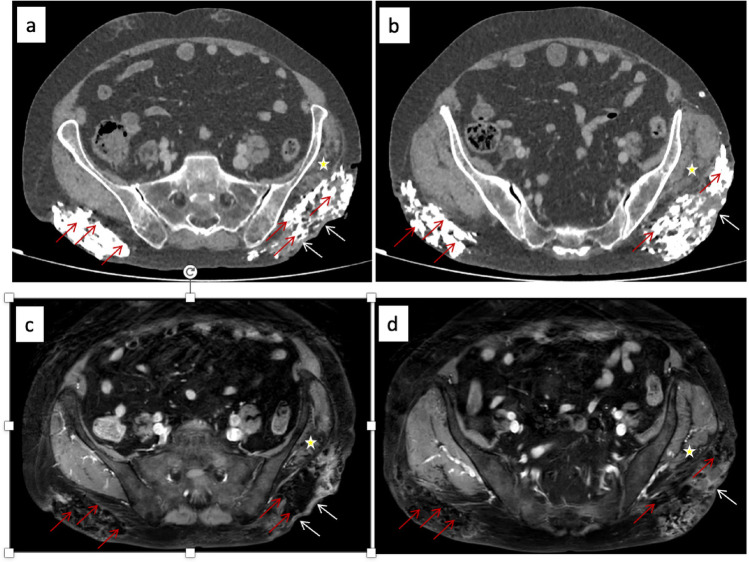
CT (a, b) and MRI (c, d) scans of the pelvis demonstrate extensive, bilateral dystrophic calcifications within the subcutaneous fat of the gluteal regions (red arrows). In the left gluteal region, imaging reveals irregular soft tissue thickening accompanied by focal skin retraction (white arrows) and probable fibrous tissue formation. Note the extensive fatty atrophy of the underlying gluteal muscles (yellow asterisks)
